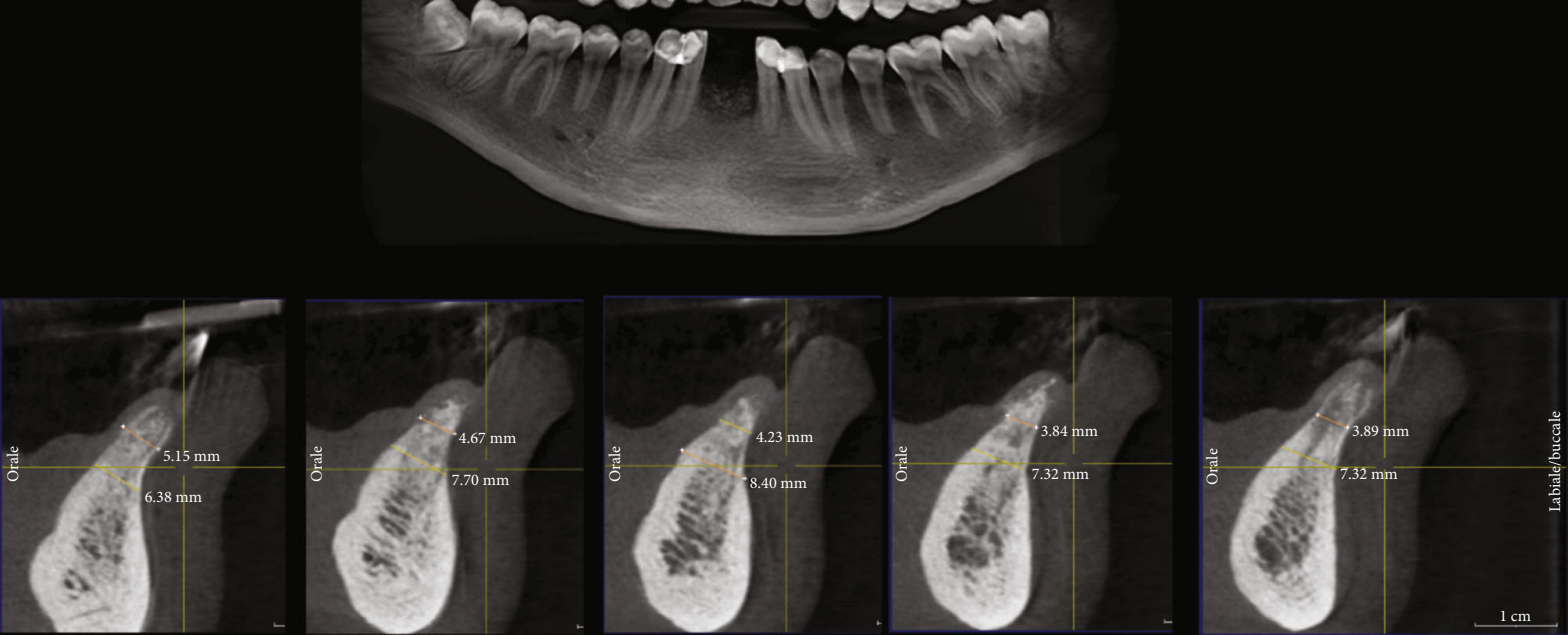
Section of CBCT scan, taken after 5 months, showing bone healing after grafting.
